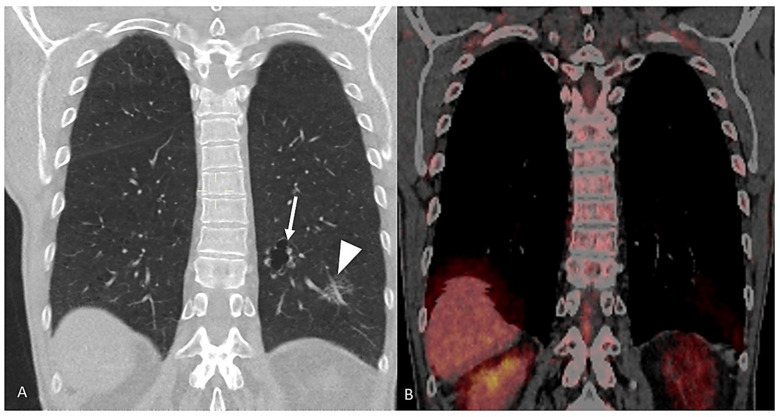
Coronal chest CT (A) shows two adenocarcinomas in the left lower lobe, one with the appearance of an atypical cyst (white arrow) and the other one with the appearance of a partially solid nodule (white arrowhead). PET-CT (B) of the same patient does not show any significant metabolic activity of the two lung nodules.
